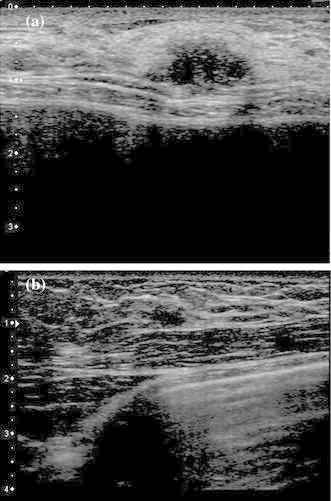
Ultrasound (US). US revealed a 15 mm hypoechoic mass with an irregular margin and rupture of the anterior borderline (a). US incidentally revealed a hypoechoic mass in the right breast (b)
